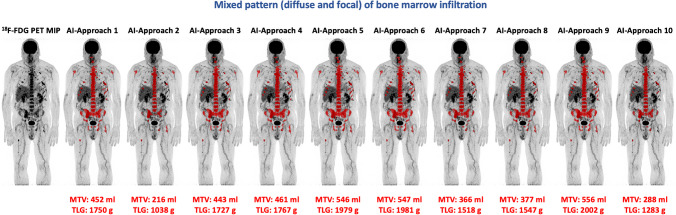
Example of the application of the AI-based software tool for automated calculation of total MTV and TLG of a MM patient with intense diffuse BM [^18^F]FDG uptake as well as multiple focal hypermetabolic lesions. The use of different tracer uptake thresholds leads to different BM segmentation patterns and, subsequently, to different whole-body MTV and TLG values
